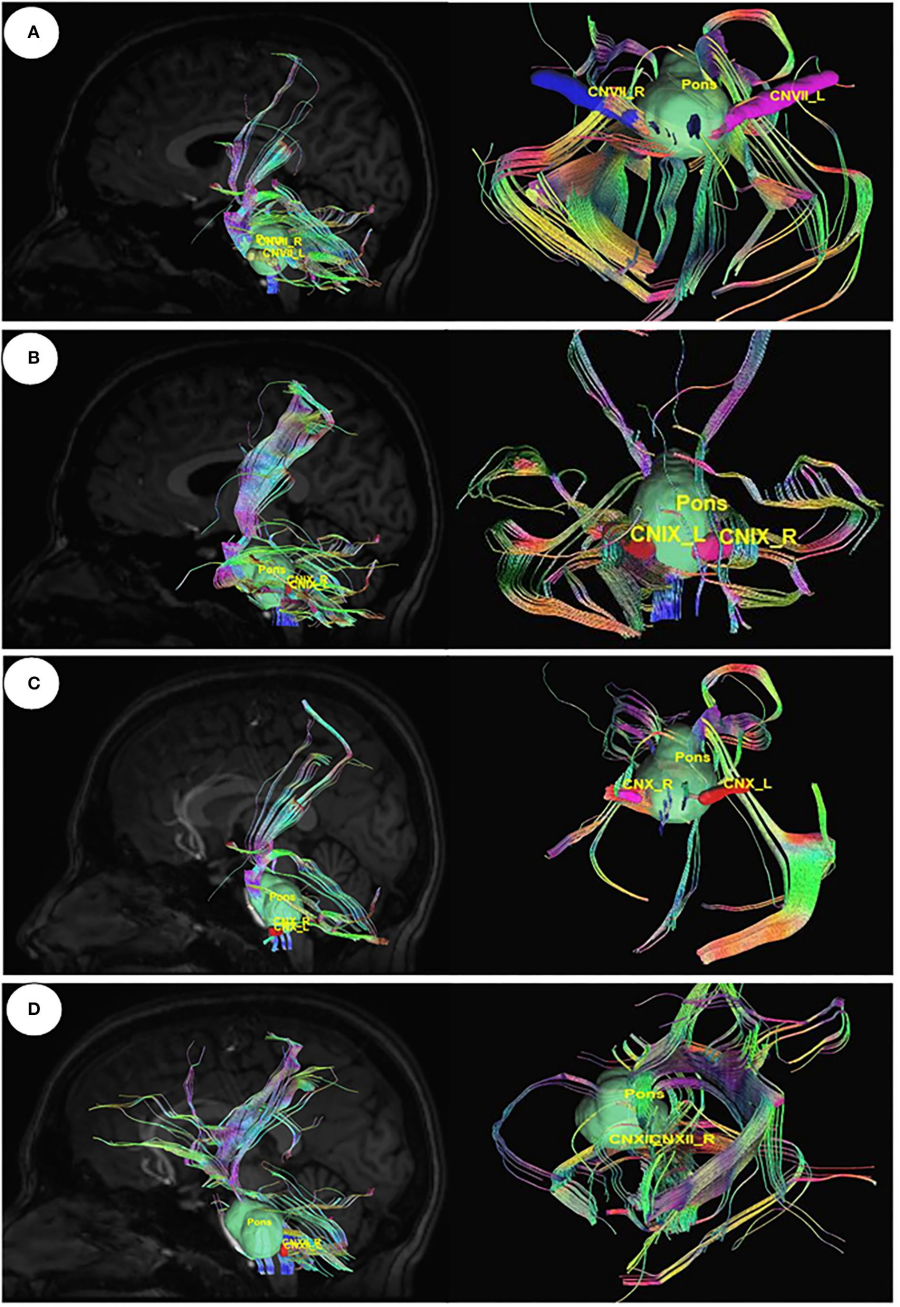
Diffusion MRI fiber tracking-connecting pons with the gustatory nerves. (A) pons to cranial nerves VII, (B) pons to cranial nerves IX, (C) pons to cranial nerves X, and (D) pons to cranial nerves XII.
